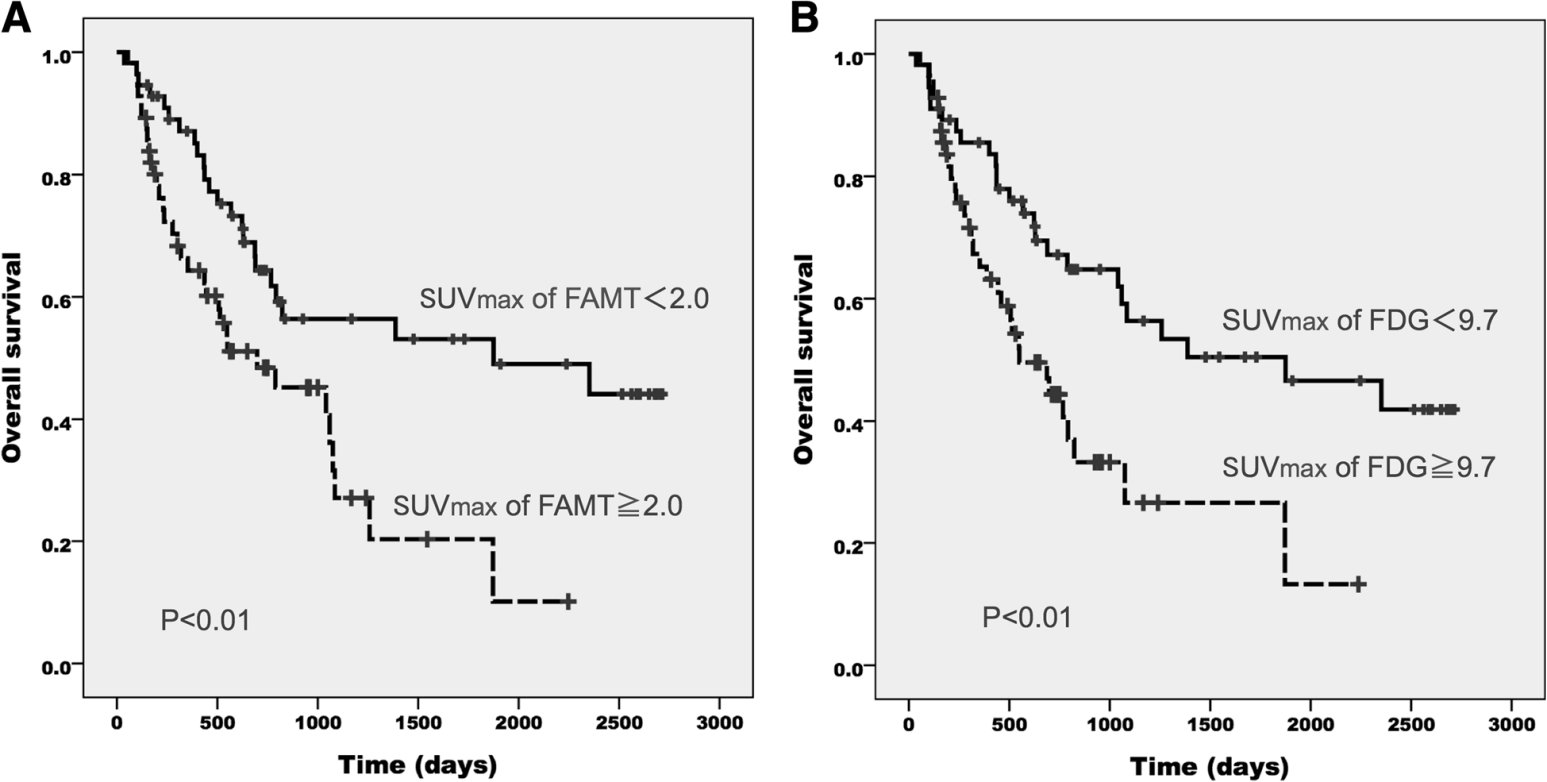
Overall survival of NSCLC patients according to the SUV_max_ of ^18^F-FAMT (a) and ^18^F-FDG (b)
